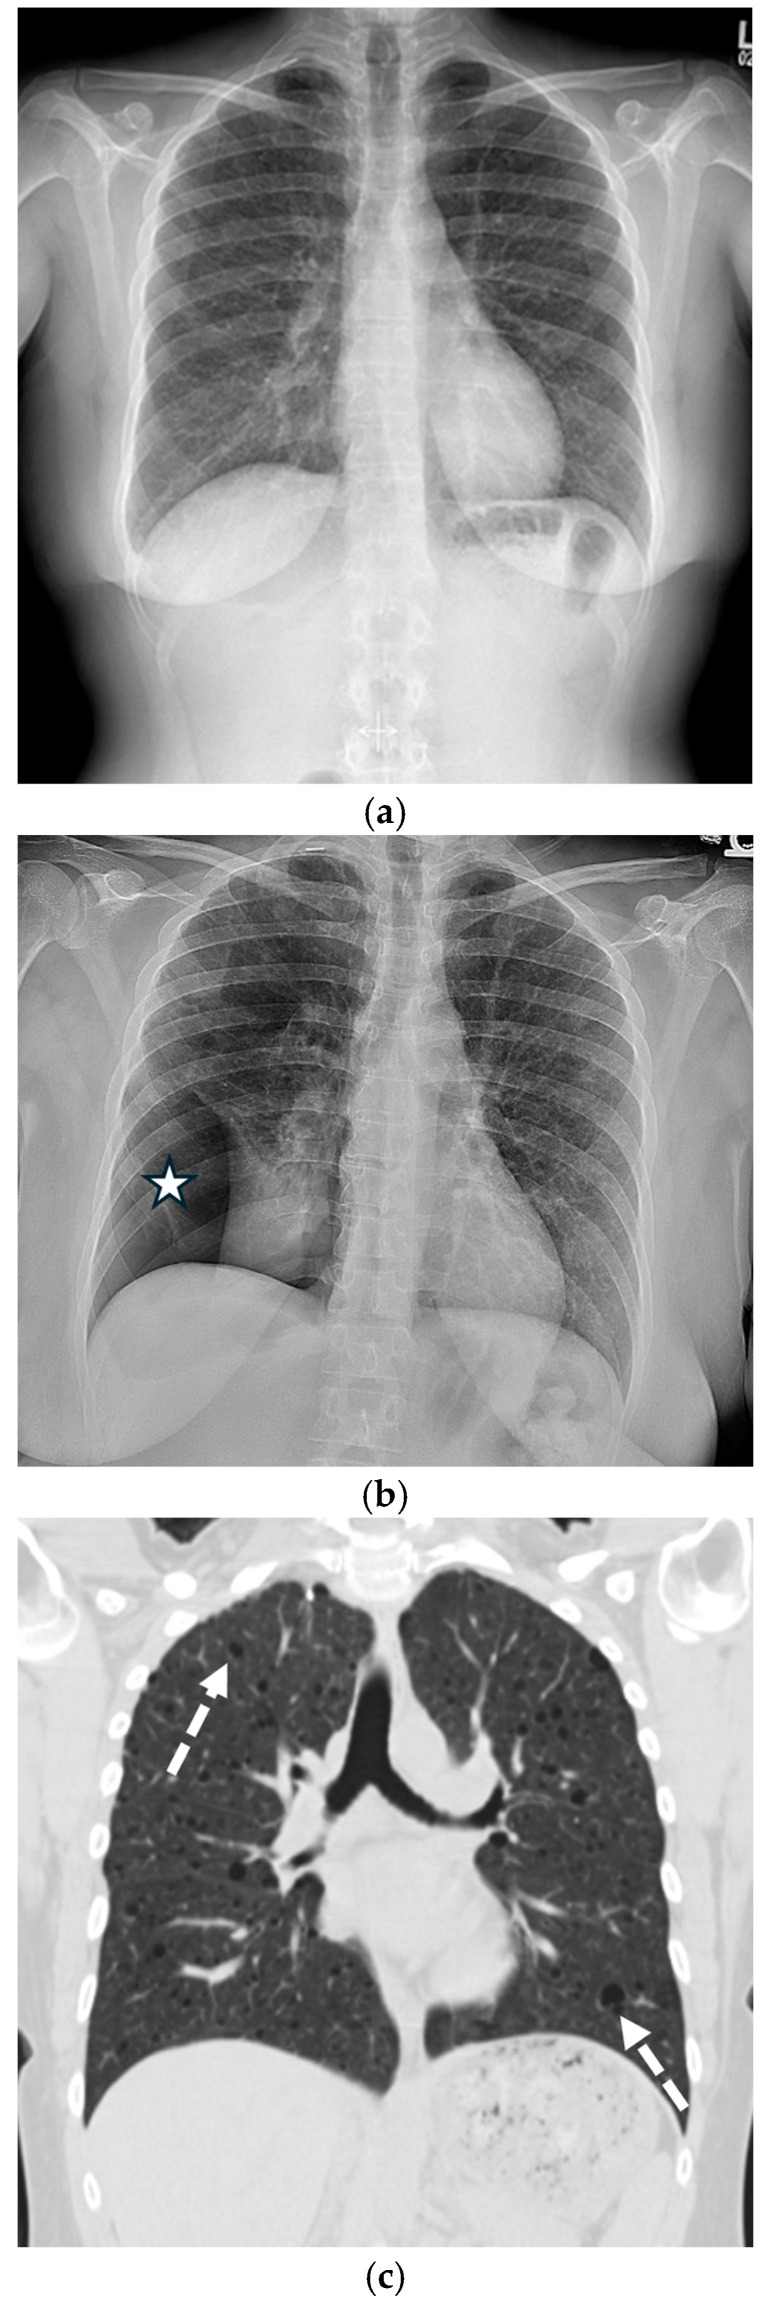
44-year-old female with LAM presents with chest pain. A frontal chest radiograph from 2 years ago (a) shows mild pulmonary interstitial prominence. Subsequent frontal chest radiograph (b) reveals a new moderate right pneumothorax (white star) with partial right-lung atelectasis. Coronal CT image in lung window (c) demonstrates multiple diffusely distributed thin-walled cysts (dashed white arrows) with normal areas of intervening lung.
